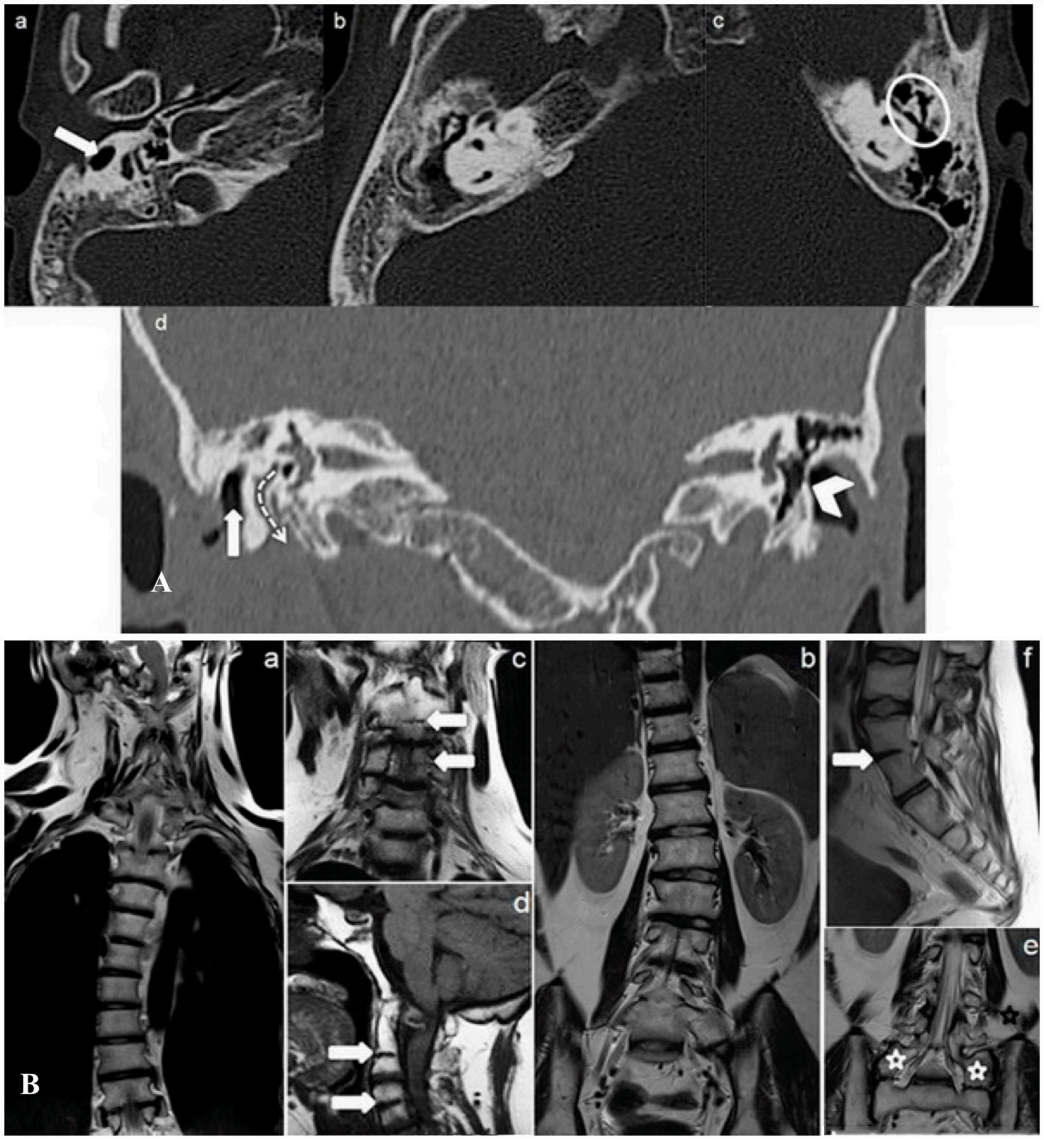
(A) Transverse (a–c) and Coronal (d) minimum intensity projection (MIP) CT temporal bone study. Right external auditory canal malformation (a) (white arrow); right chronic otitis media (b) (white star); ankyloses of left ossicular chain (c) (white circle); right widening of facial canal (third segment) (dotted arrow) and partial calcification of left tympanic membrane (arrowhead) (d). (B) MR Coronal T2 (a–c, e), Sagittal T1 (d), and Sagittal T2 (f) weighed sequences whole spine study. The study shows, besides rotoscoliosis (a, b), cervical (C 2–C 3, C 4–C 5) (d, f) and lumbar (L 4–L 5) vertebral fusion (white arrows), and L 5 bilateral sacralisation (e) (white stars) (KFS type II malformation).
